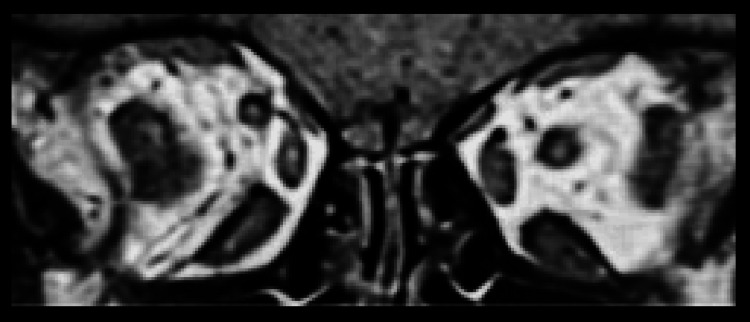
MRI findings just before the third surgery. MRI, magnetic resonance imaging
